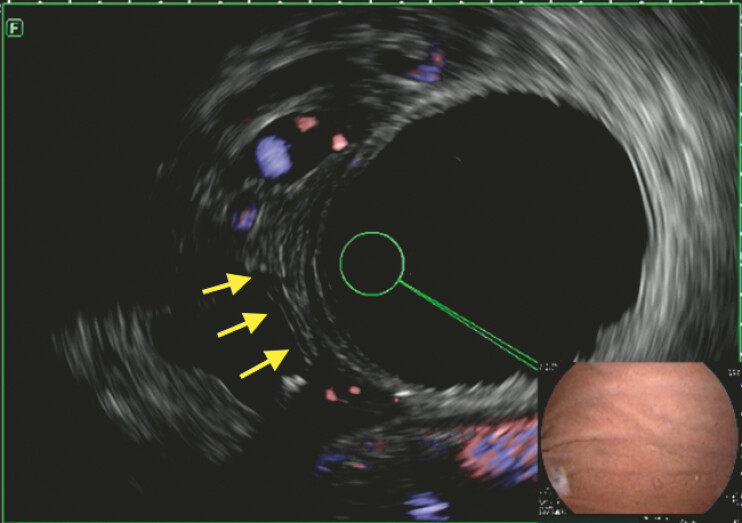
EUS examination with Doppler without major Doppler activity within septum. Abbreviation: EUS, endoscopic ultrasound.
